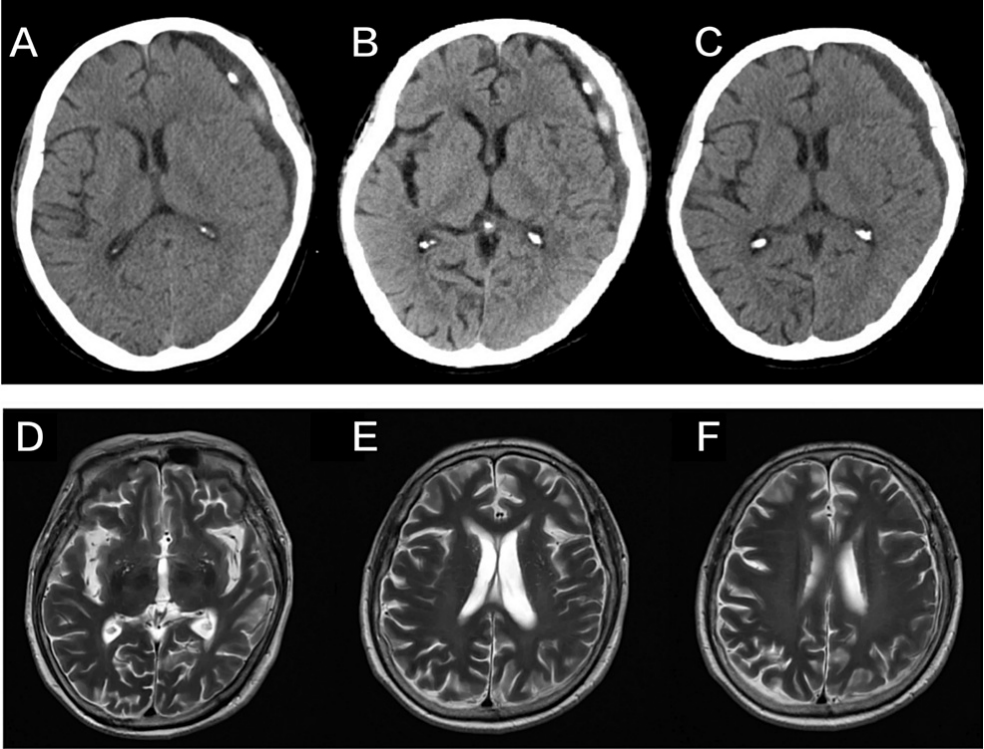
Axial brain images after the second operation. Upper row (A, B, C): Postoperative CT showing the changes in the chronic subdural hematoma. A: On day two, residual hematoma and a high-density shadow were detected. B: On day four, the residual hematoma and high-density shadow persisted even after the administration of 20,000 units of urokinase. C: On day 13, no significant mass effect or high-density shadow was observed. Lower row (D, E, F): Postoperative MRI (day 113) demonstrating normal brain structure without evidence of any subdural fluid collection.
